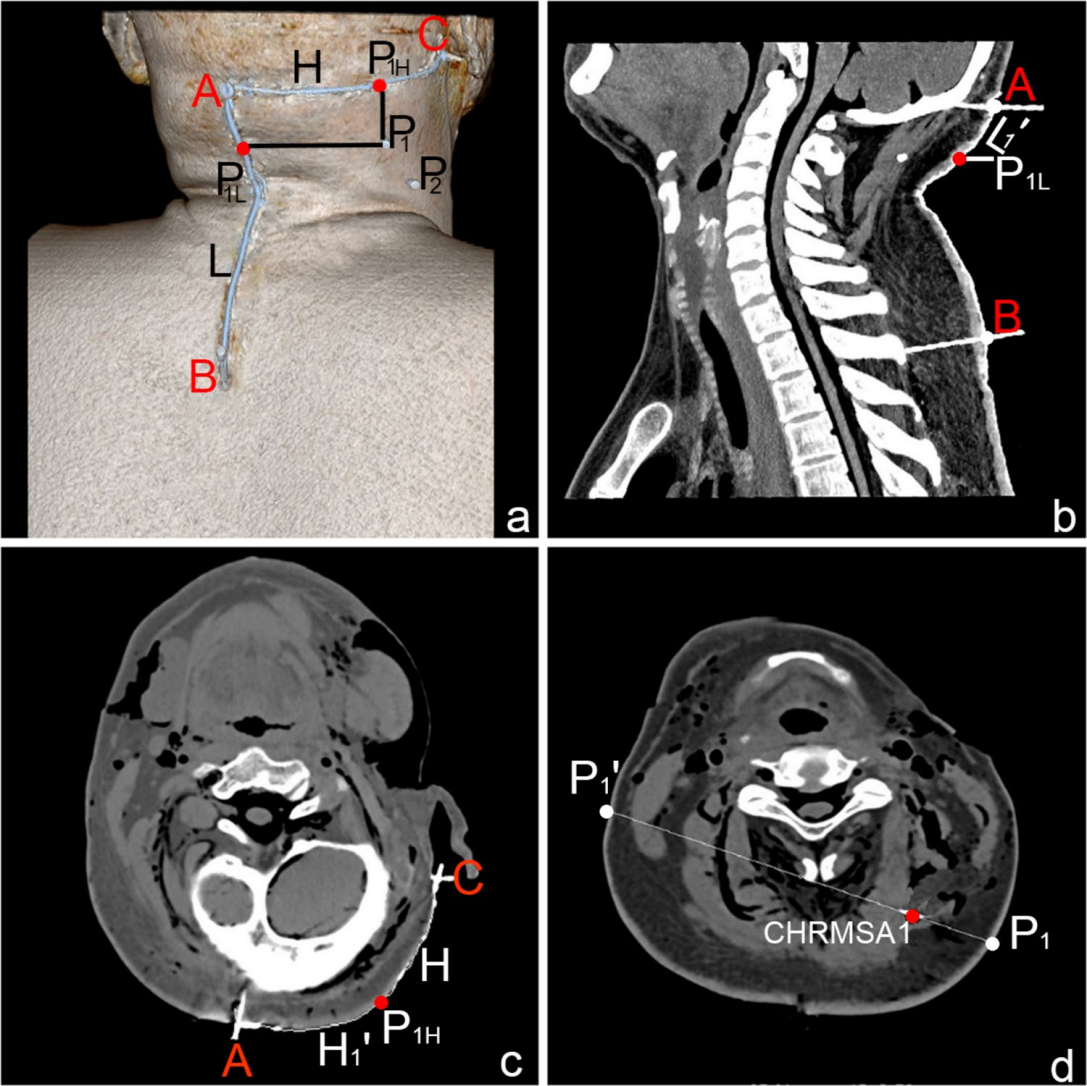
Spiral CT image of CRHMSA localization of the right splenius capitis muscle. a Body surface projection position of the CRHMSA and its reference line. A = external occipital protuberant, B = spinous process of the third thoracic vertebra, C = mastoid process, P_1_ = body surface projection point of the CRHMSA in the splenius capitis muscle, P_2_ = body surface projection point of the CRHMSA in the splenius cervicis muscle, AB = L, AC = H; A-P_1_L = L_1_', A-P_1_H = H_1_'. b Measurement of the length of the L and L_1_' lines on the sagittal section through line AB. c Measurement of the length of the H and H_1_' lines on the cross-section through line H. d Measurement of the depth of CRHMSA_1_ through point P_1_
